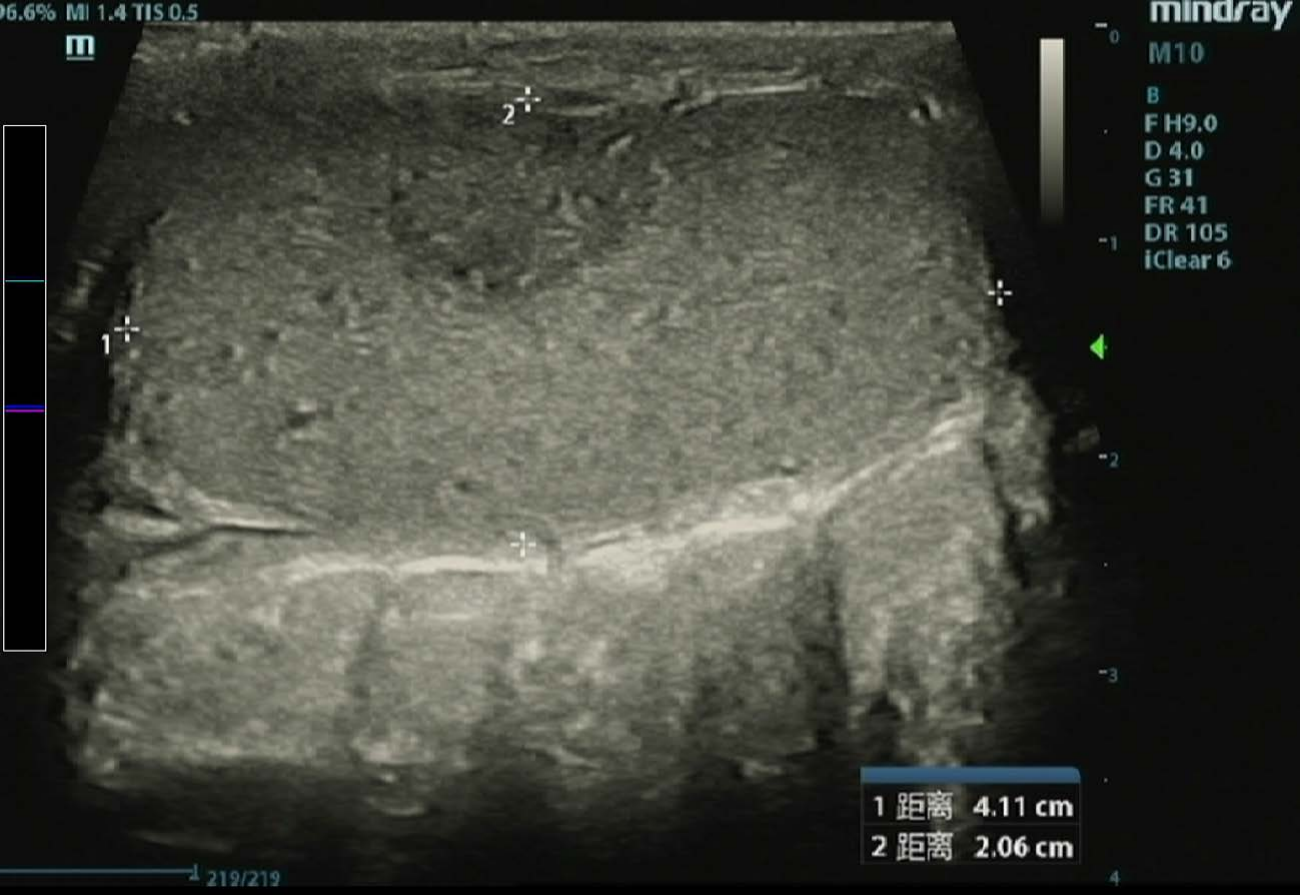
Ultrasound shows heterogeneous hypoechoic area with unclear boundary.
